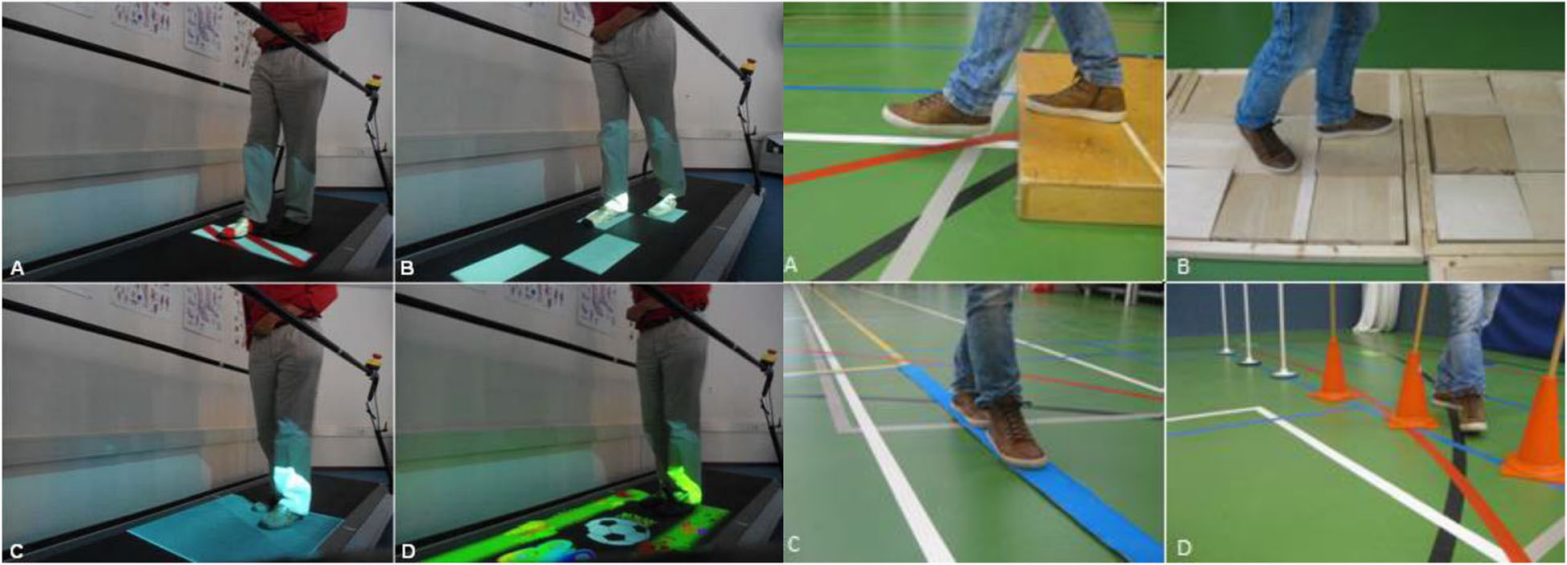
Snapshots of the two interventions aimed at improving walking adaptability. (Left) Walking-adaptability exercises of treadmill-based C-Mill therapy (CT); A obstacle avoidance, B goal-directed stepping, C gait acceleration and deceleration, and D a functional and interactive walking-adaptability game. (Right) Obstacle course of the overground FALLS program (FP); A obstacle avoidance, B walking over uneven terrain, C tandem walking, and D slalom walking
